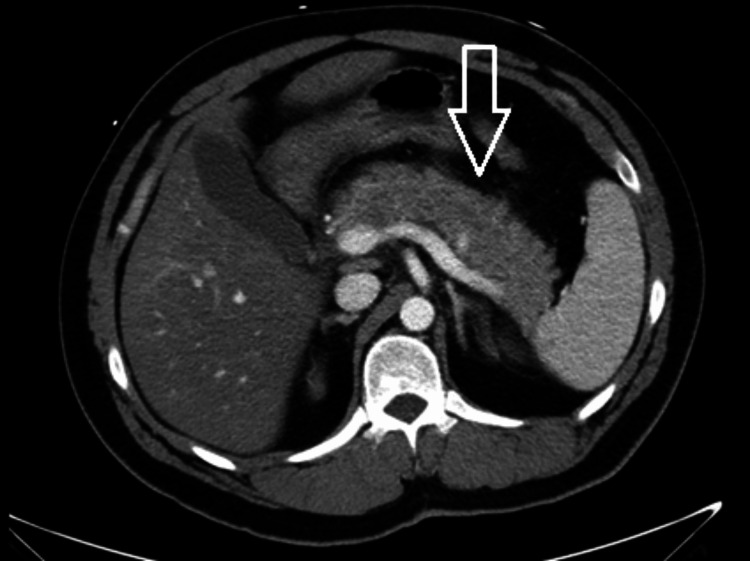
Contrast-enhanced CT of the abdomen and pelvis on hospital day one Representative axial CT image showing acute interstitial pancreatitis with peripancreatic inflammatory change on initial imaging. The arrow indicates an area of peripancreatic inflammatory change.
